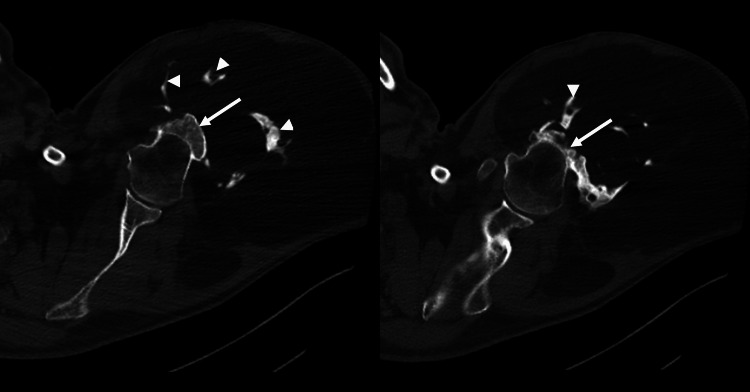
Axial bone window CT. A large lipomatous lesion adjacent to the left humerus is visible. An irregular osseous excrescence (arrow) is noted, lacking continuity with the humeral bone marrow. Additionally, multiple other foci of ossification within the lesion are observed (arrowheads).
